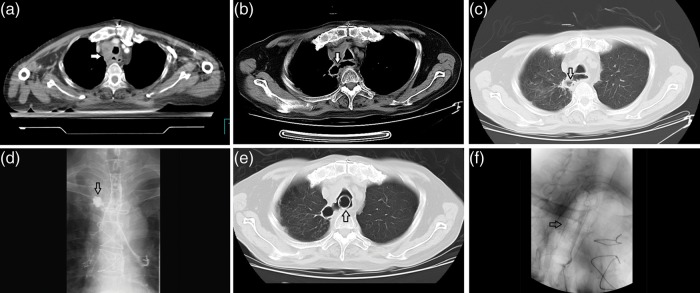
A patient with esophageal cancer who received radiotherapy after exploratory thoracotomy, (a) CT image when started the RT, the arrow head showed an extracapsular LN invading the esophagus, (b) CT image (mediastinal window) of the esophageal perforation (the arrow head indicates the actual communication of an air-filled esophagus with an adjacent mediastinal or paramediastinal air–fluid collection), (c) the perforation on lung window, (d) the leakage of iodine on iodine examination, (e) CT image after the placement of a self-expandable metallic stent (the arrow head indicates the stent), and (f) an X-ray after the placement of a stent (the arrow head indicates the stent).
